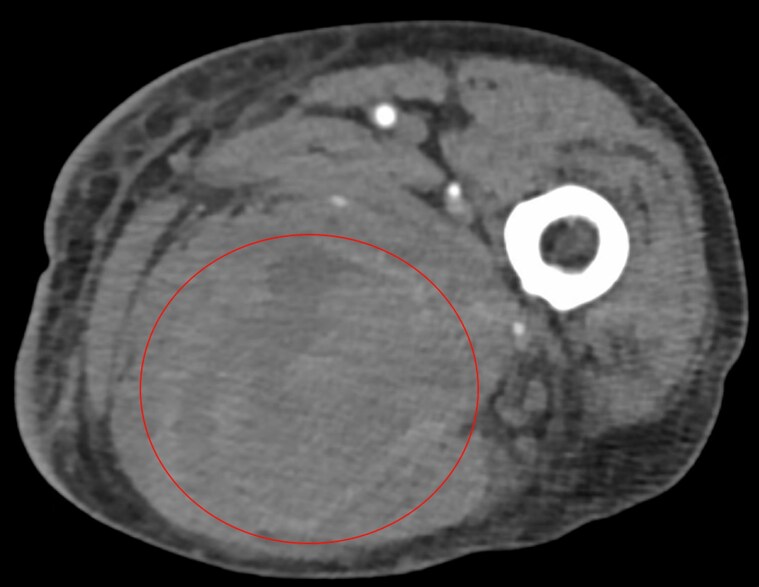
The CT angiographic scan of the left lower extremity reveals the haematoma encircled by the red circle.
